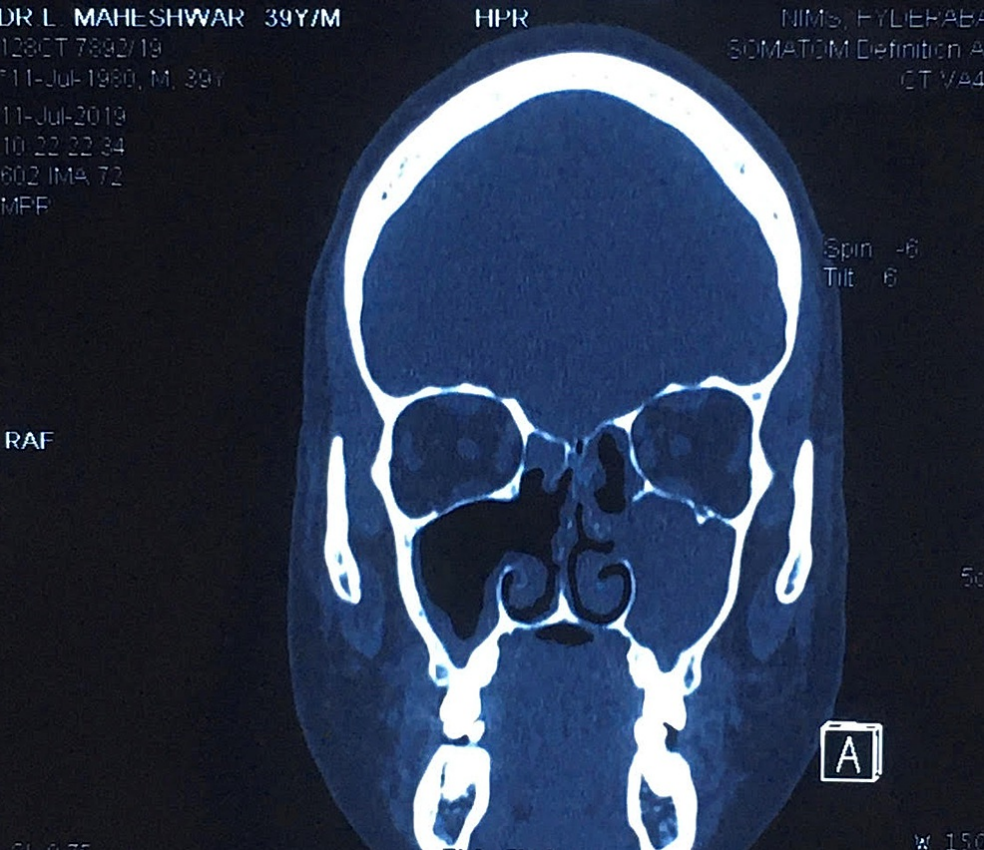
Coronal CT image of paranasal sinuses done in 2019 showing postoperative status with pansinusitis with bilateral mucosal thickening and collection of pus in the left maxillary sinus. CT: computed tomography
